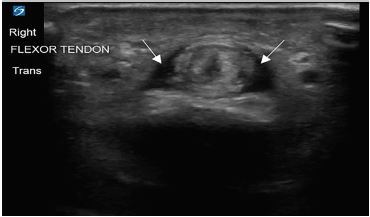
Transverse ultrasound view of the right middle finger. The image displays anechoic fluid (white arrows) surrounding the flexor tendon within the tendon sheath.
